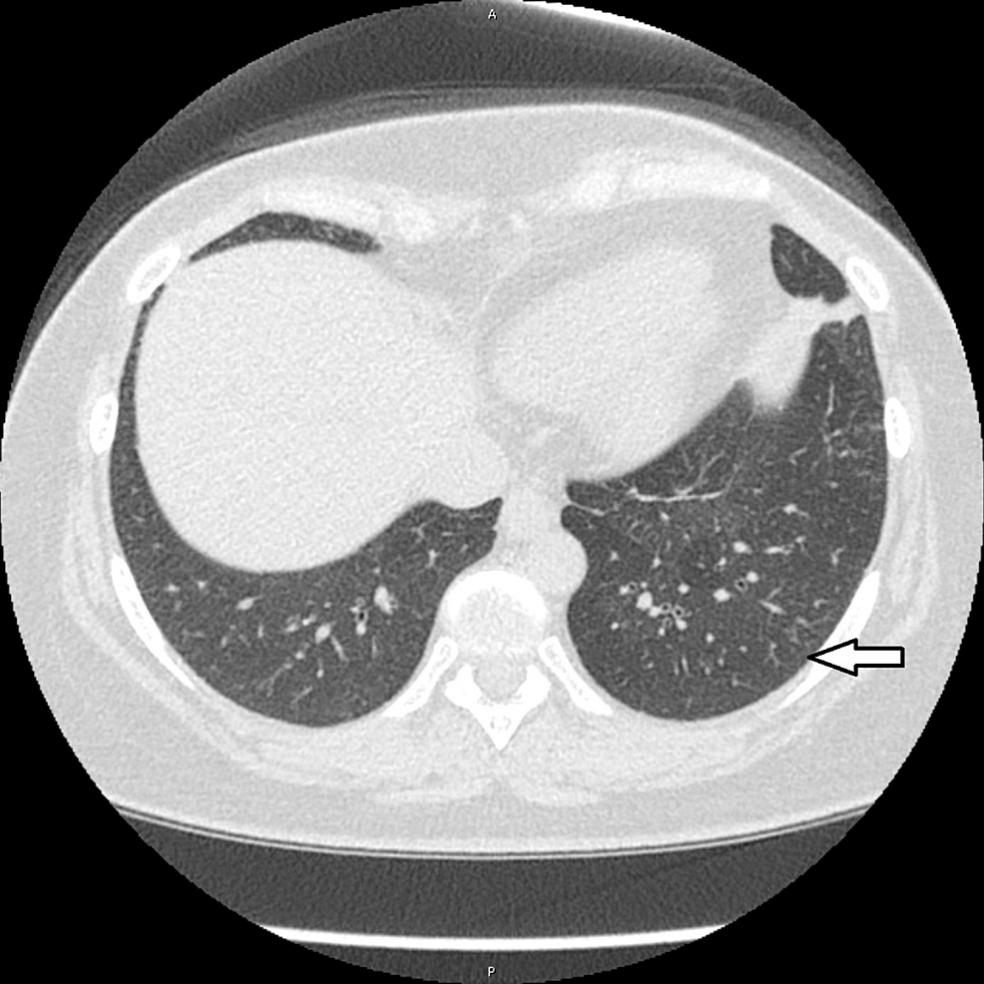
CT of the chest. Lung window. Lung lower lobes. The improvement of the left lower lobe nodules (arrow). Compare with Figure 3.
